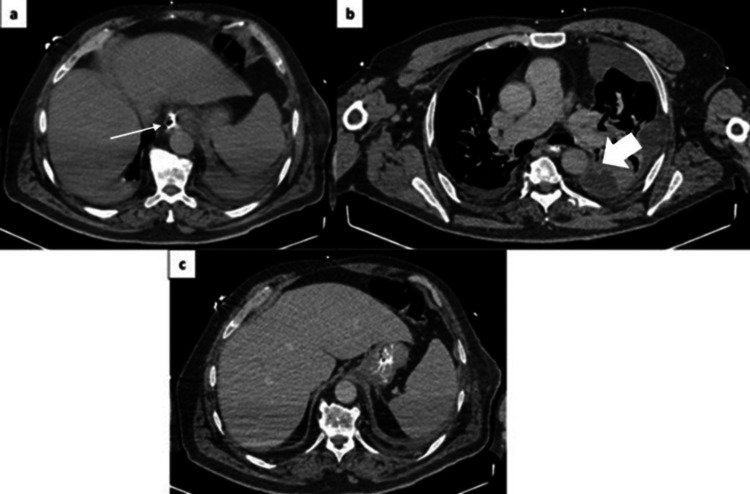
(a) Non-contrast CT showing the presence of hyperdense material in the region of the esophageal rupture, related to the conservative treatment performed by endoscopy (thin arrow); (b) and (c) After administration of oral contrast, passage of contrast into the gastric cavity is noted without evidence of leakage. Additionally, persistence of the bilateral pleural effusion, partially loculated on the left, is seen (thick arrow). CT, Computed Tomography
